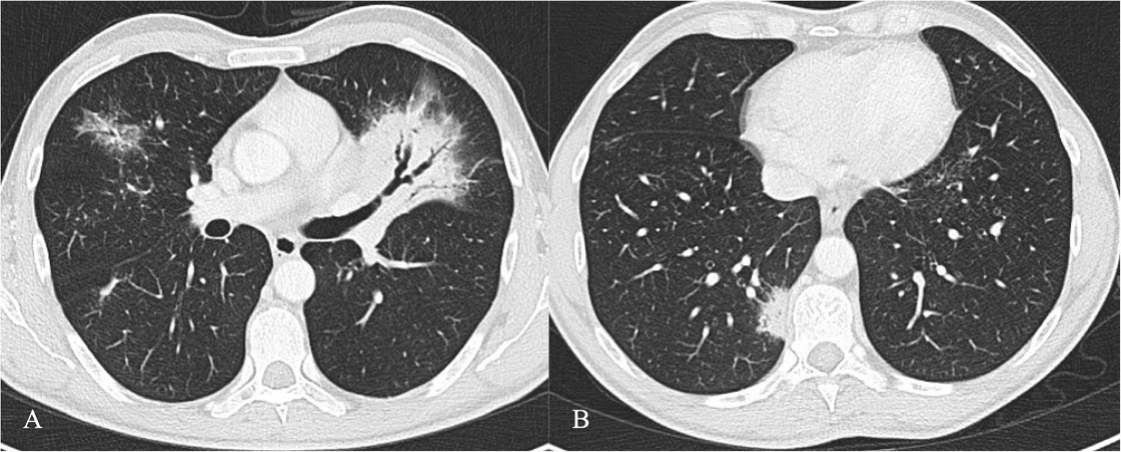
COP pattern in a 53-year-old man. HRCT images show characteristic peripheral and bronchocentric consolidations. The “air bronchogram sign” is clearly recognisable in A. Tendency to migration and changing location are also typical imaging findings of COPincrease of consolidations over several weeks after antibiotic therapy;ground-glass opacities with tendency to migration, change of location and size;rarely a mass or nodule that may cavitate or reproduce the typical appearance of an “atoll sign” (Fig. 15);
